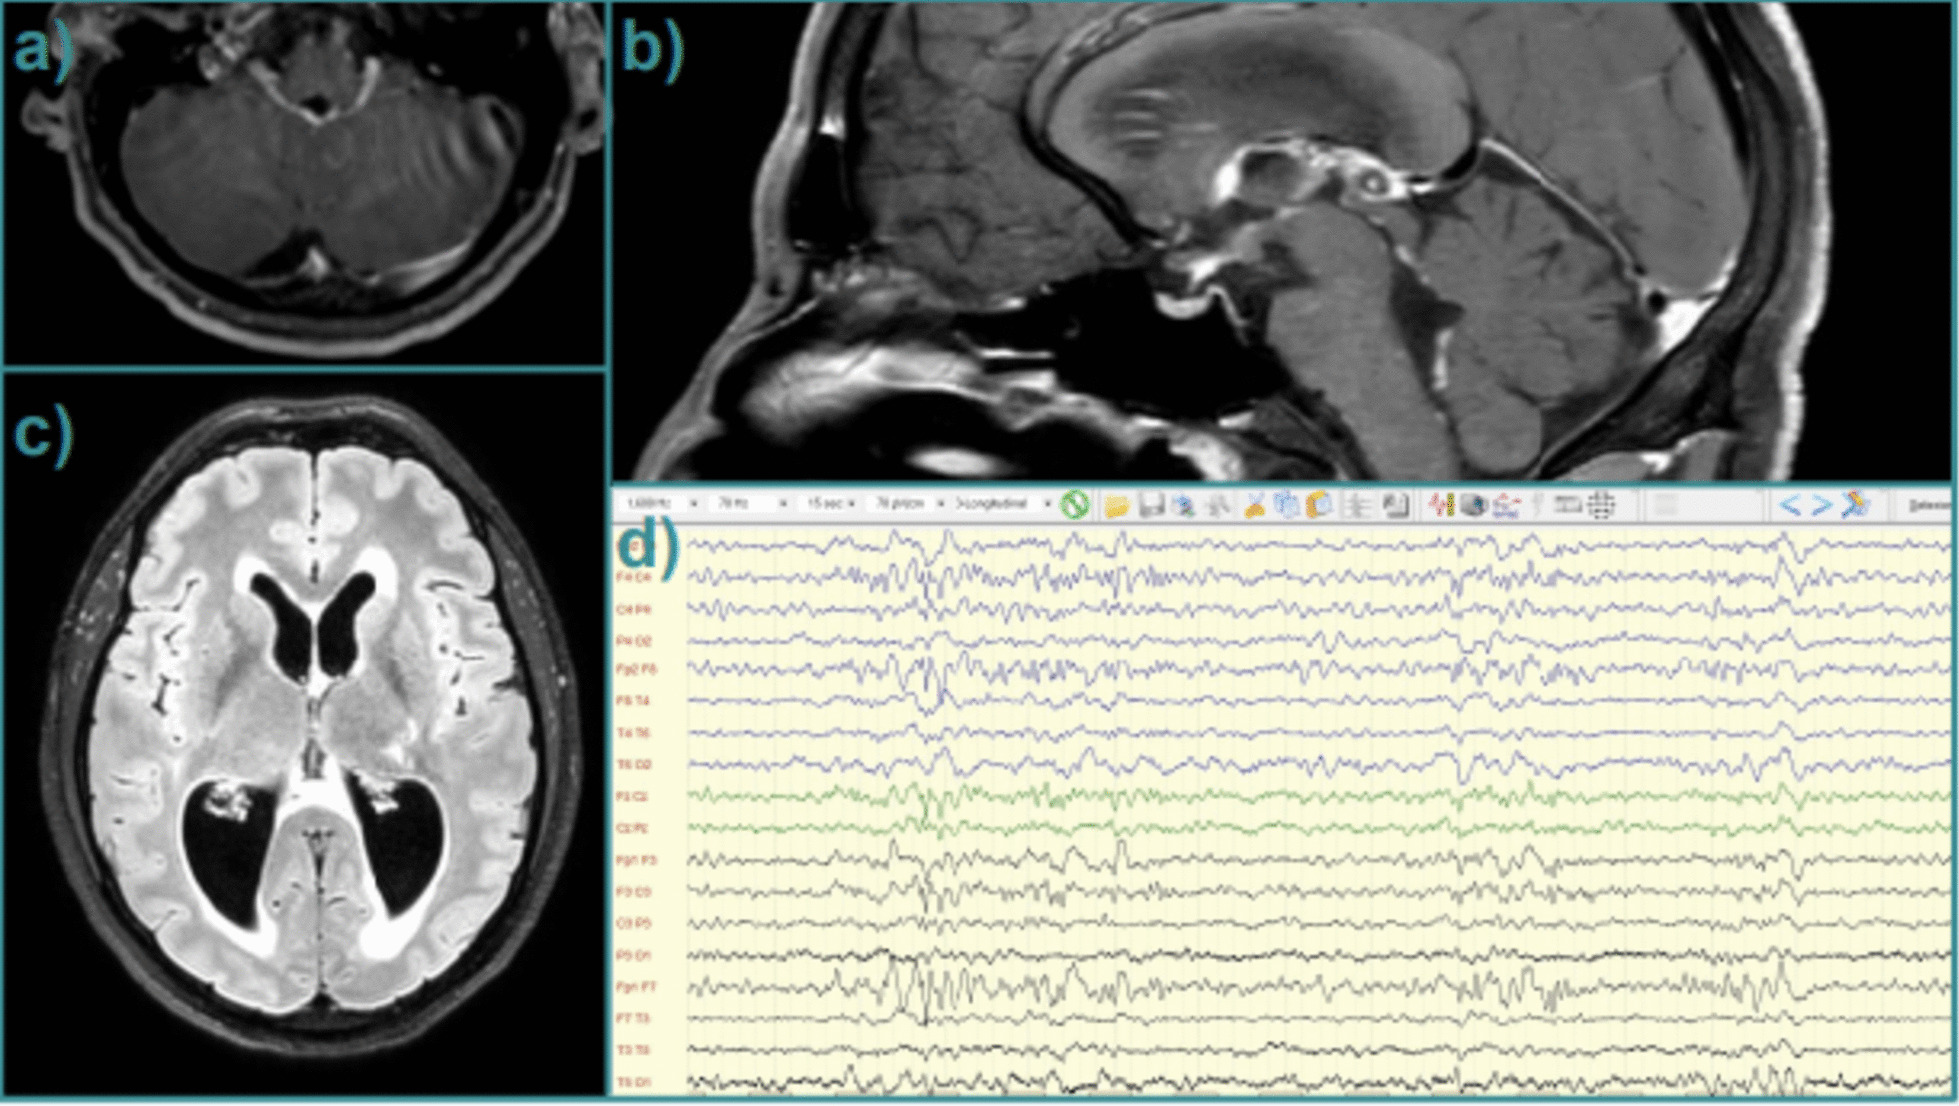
Main examinations performed after clinical worsening; brain MRI shows biventricular hydrocephalus and contrast enhancement of ependyma at the cerebral aqueduct (III and IV ventricles, a-c). Electroencephalogram shows intercritical epileptic discharges (d)
